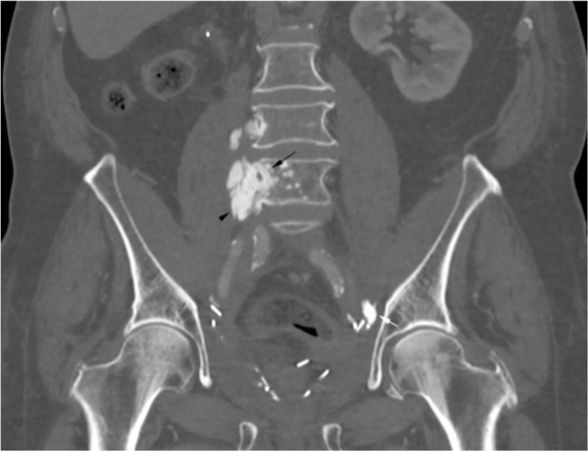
Coronal reformat of a CT shows the multifocal ossifications along the retroperitoneal space (black arrowheads) as well as the intravertebral extension (black arrow). A calcified nodule is seen in the left inguinal area (white arrow)
